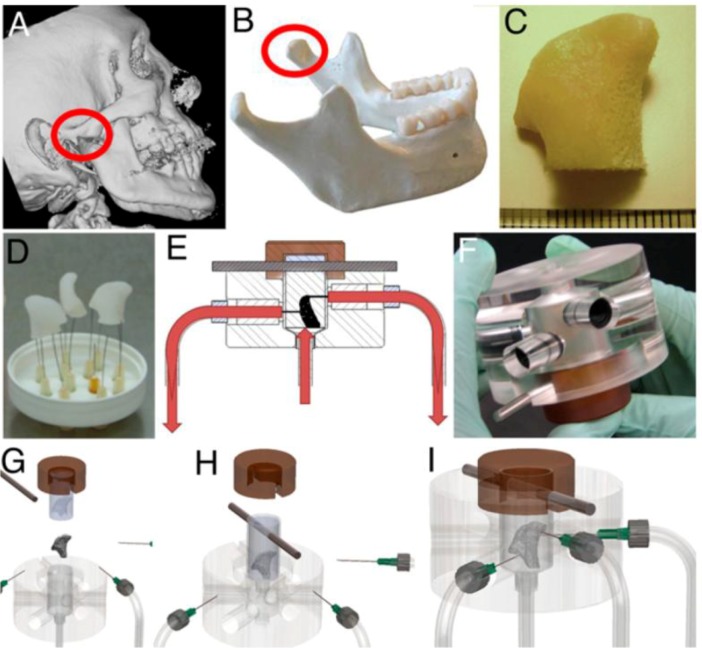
Tissue engineering of anatomically shaped bone grafts using 3D flow. (A,B) computed tomography (CT) images were used for the reconstruction of exact geometry of temporomandibular joint (TMJ)condyles. (C) Scanned data were used tomachine TMJ-shaped scaffolds from fully decellularized trabecular bone. (D) Image of produced scaffolds. (E) Human mesenchymal stem cells (hMSCs) were statically cultured for 1 week in the scaffolds, and then theperfusion was applied for additional 4 weeks.(F) Image of the perfusionbioreactor. (G–I) Keysteps in bioreactor assembly. Reprinted with permission from the National Academy of Sciences of the United States (Grayson * et al.* [60]).
